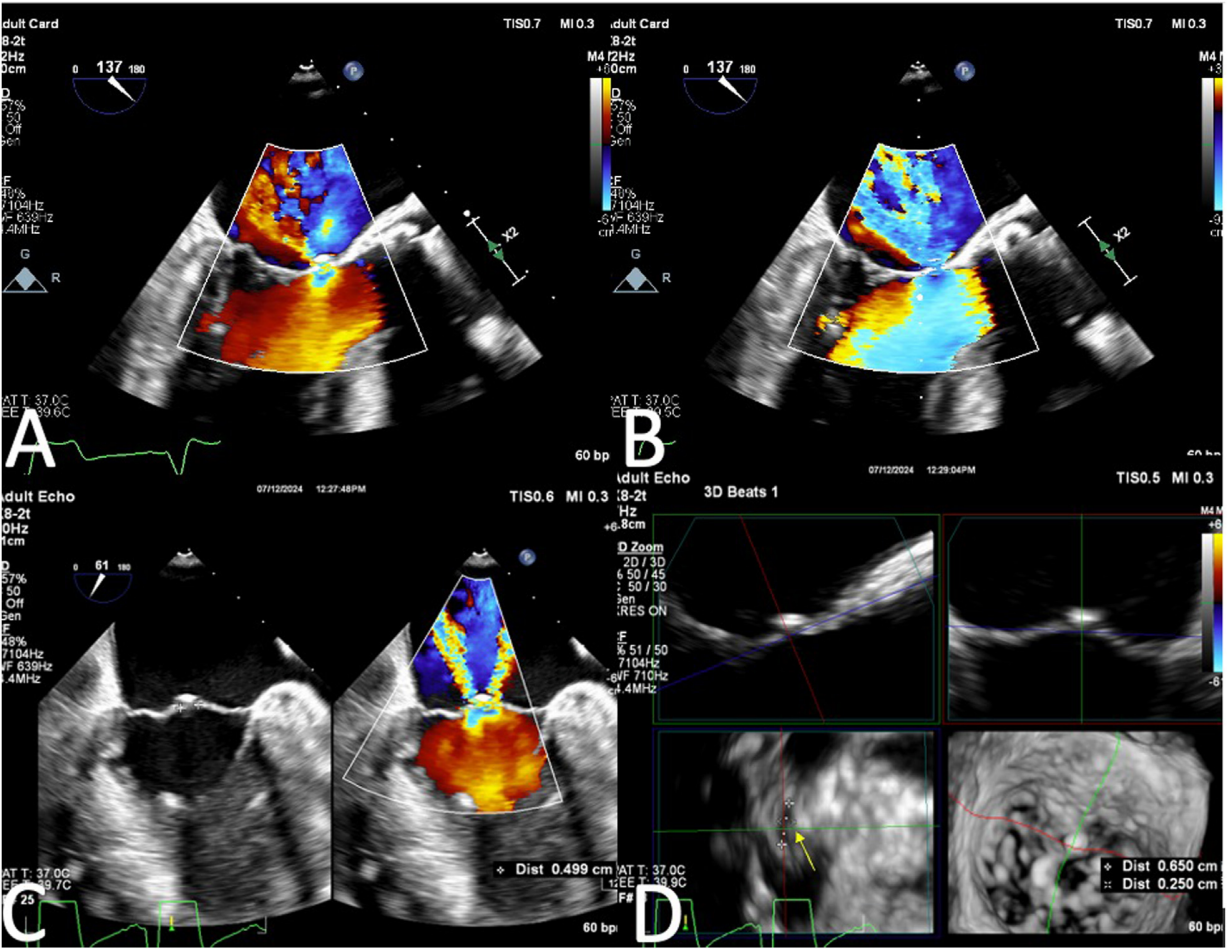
(A) Transesophageal echocardiogramleft ventricular outflow tractview demonstrating perforation of the basal anterior mitral valve leaflet. (B) PISA radius 1 cm through perforation consistent with severe mitral regurgitation. (C) 2D imaging showing the width of the perforation at 0.5 cm. (D) Three-dimensional multiplanar reconstruction sizing of the perforation at 0.65 cm × 0.25 cm (yellow arrow).
